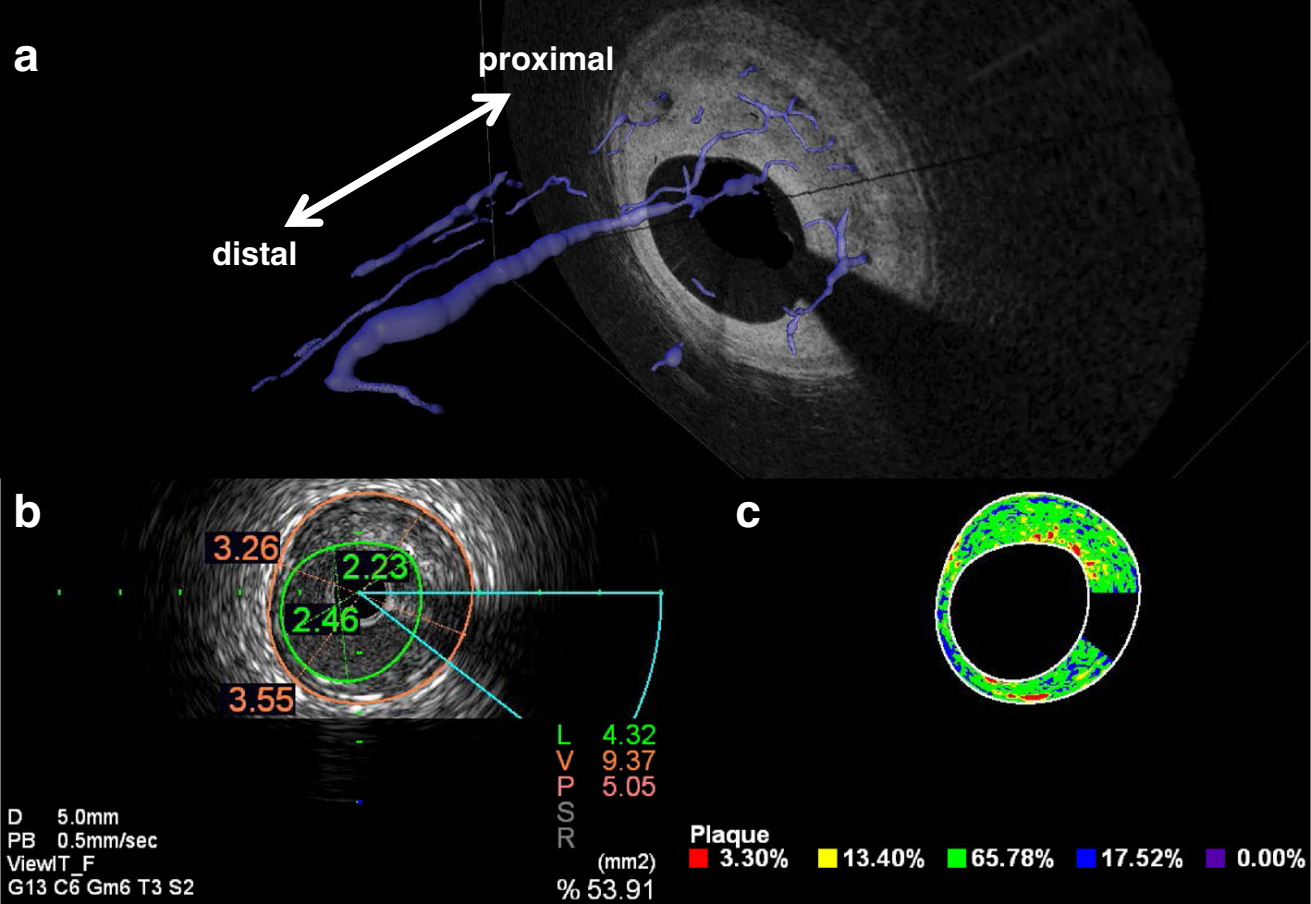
The intra-plaque NVMC was traced automatically using a three-dimensional visualization system, and was reconstructed in three dimensions (Fig. 3a). The iso-echoic eccentric plaque on gray-scale intravascular ultrasound (Fig. 3b) was mainly composed of fibrous tissue (Fig. 3c)
